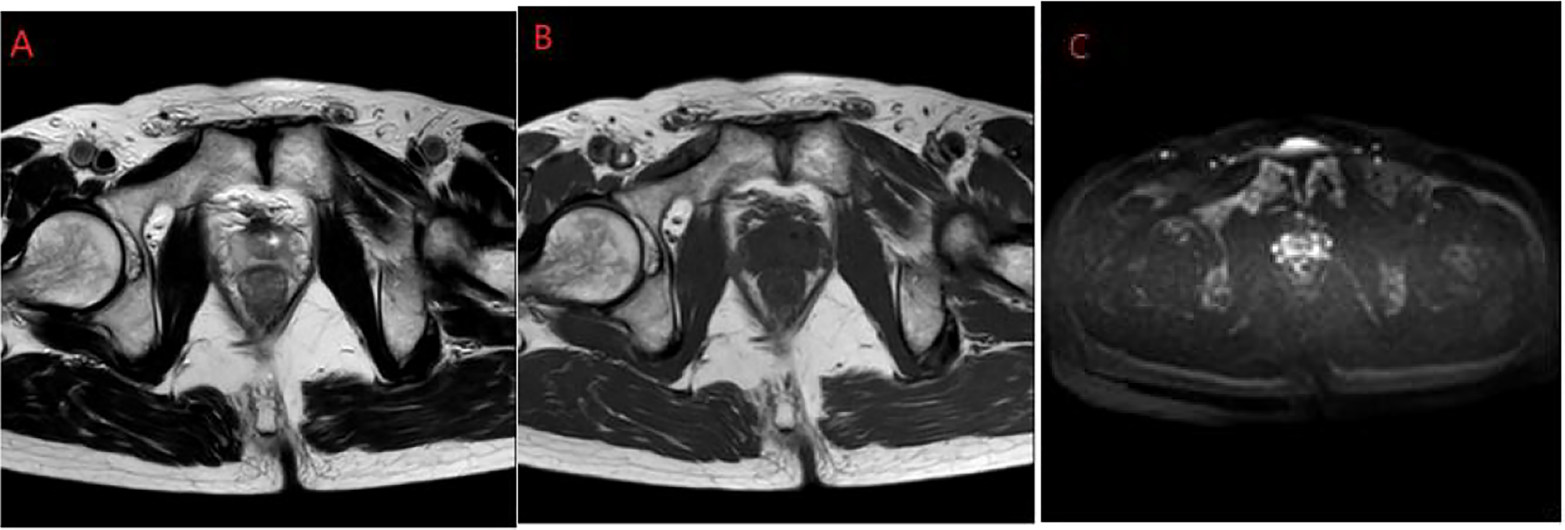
Prostate MRI. (A) Slightly longer T2; (B) Slightly longer T1; (C) Slightly high signal on DWI. MRI, magnetic resonance imaging; DWI, diffusion-weighted imaging.
